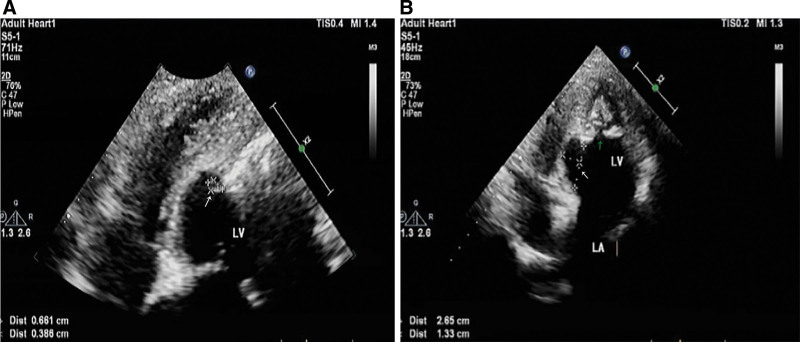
Echocardiography showing ventricular thrombus (A, white arrow), ventricular septal aneurysm (B, white arrow), apical hypertrophy and calcification (B, green arrow).
